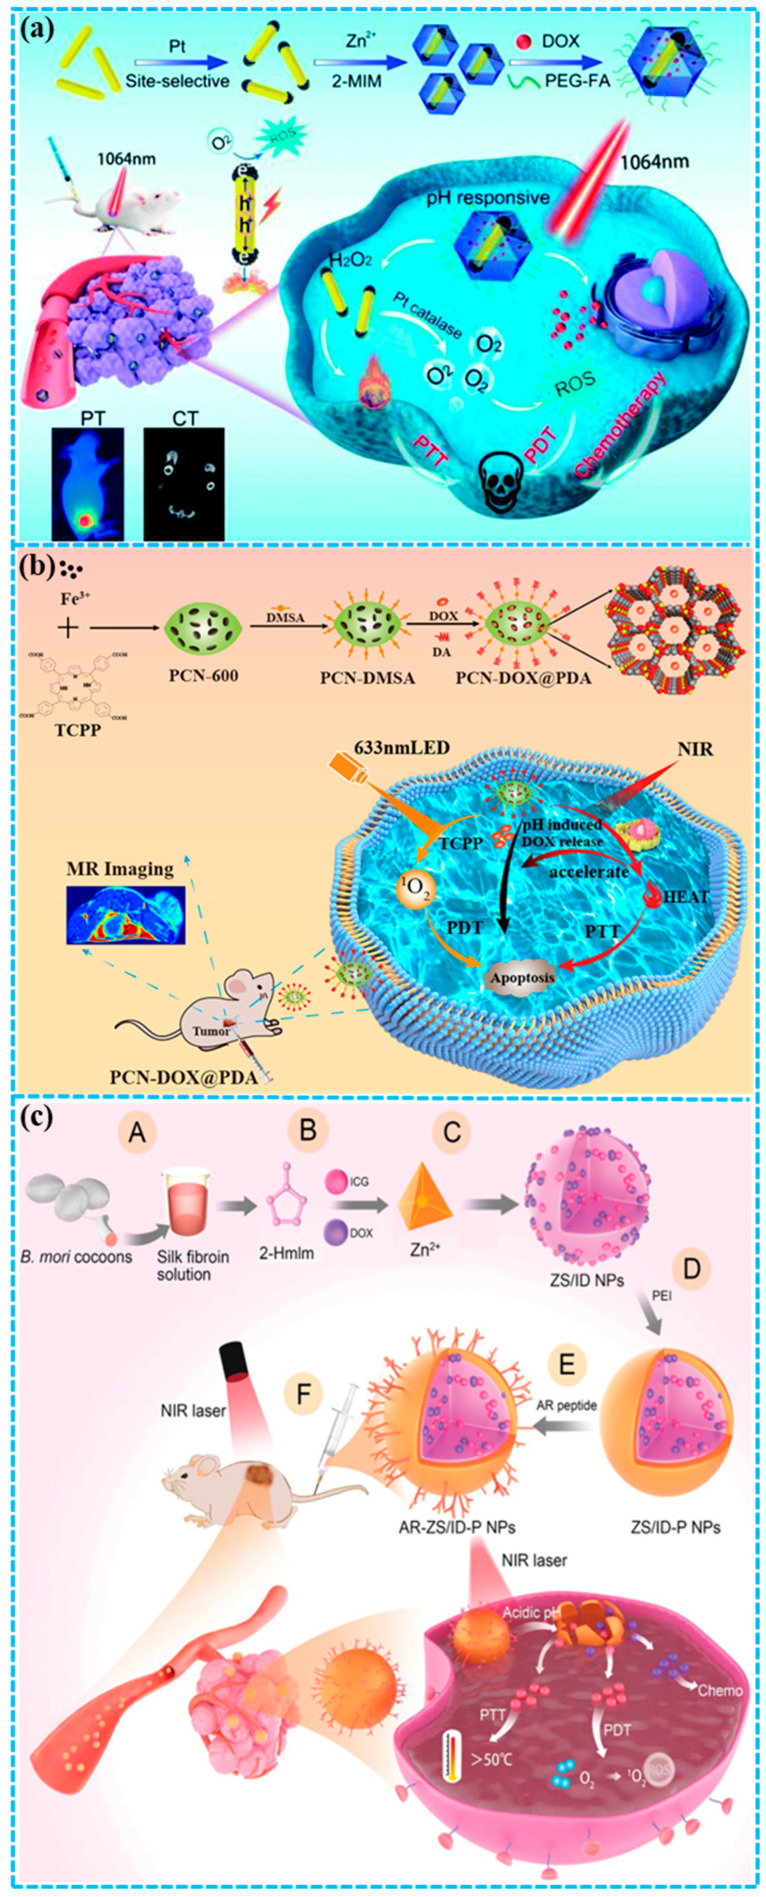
(a) Construction of a DOX-Pt-tipped Au@ZIF-8 nanoplatform for PT/CT imaging-guided combinatorial CT/phototherapy of breast cancer under a single NIR-II laser [96]; (b) preparation of PCN-DOX@PDA for MRI-guided synergistic CT/phototherapy of breast cancer [97]; (c) fabrication of AR-ZS/ID-P NPs for target peptide-guided synergistic CT/phototherapy of breast cancer [98]. Reproduced with copyright permission from Refs. [96,97,98].
